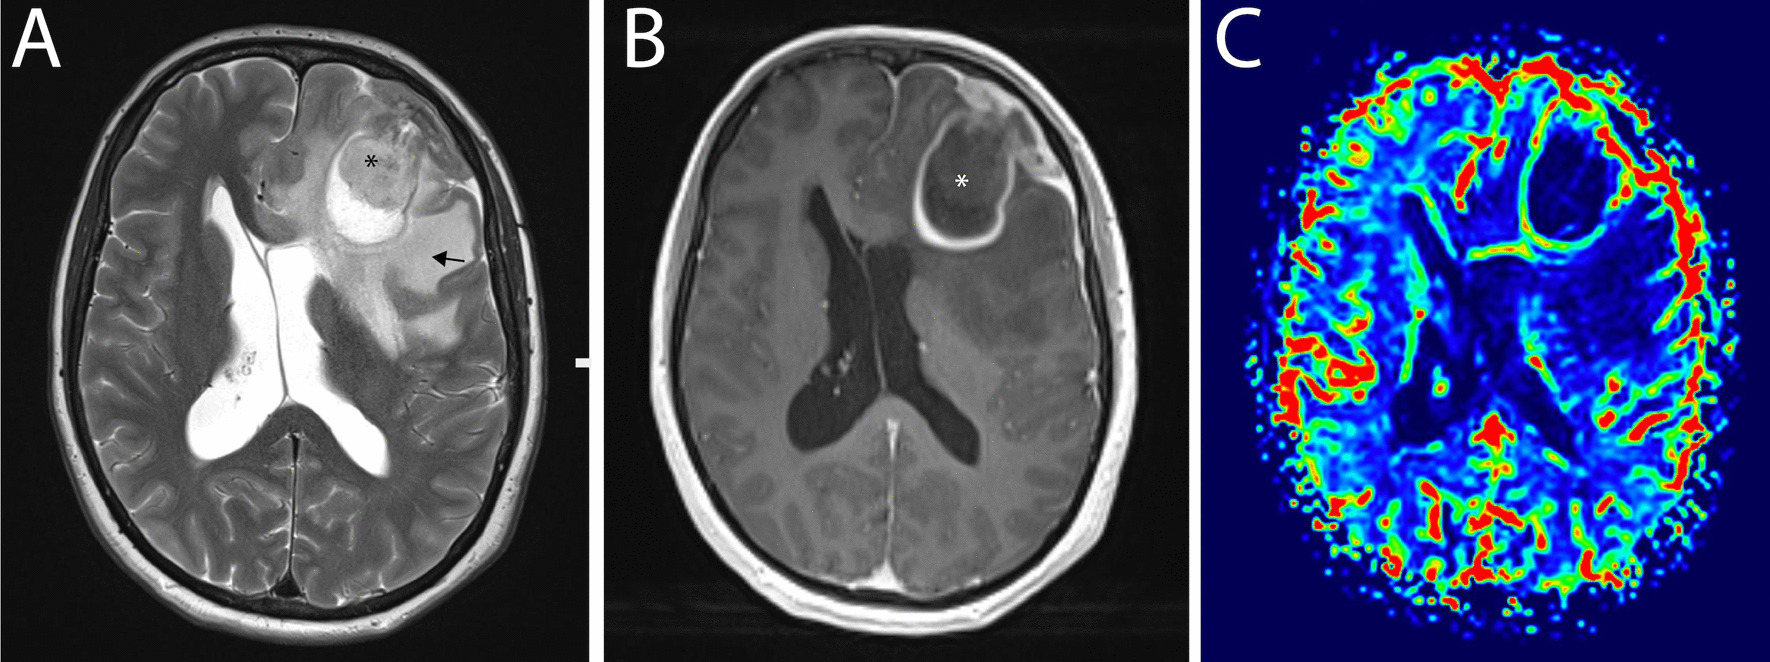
A Axial T2-w images show a cystic frontal lobe mass (asterisk) with evidence of significant surrounding vasogenic edema (black arrow). Brain compression and midline shift are evident on MRI. B Axial post-contrast T1-w images show the contrast-enhancing mass lesion (asterisk) throughout the left frontal with fairly well-encapsulated appearances. C Contrast-enhanced MRI perfusion imaging indicates high perfusion (green–red) in areas of viable tumor compared with baseline brain perfusion (black-blue)
